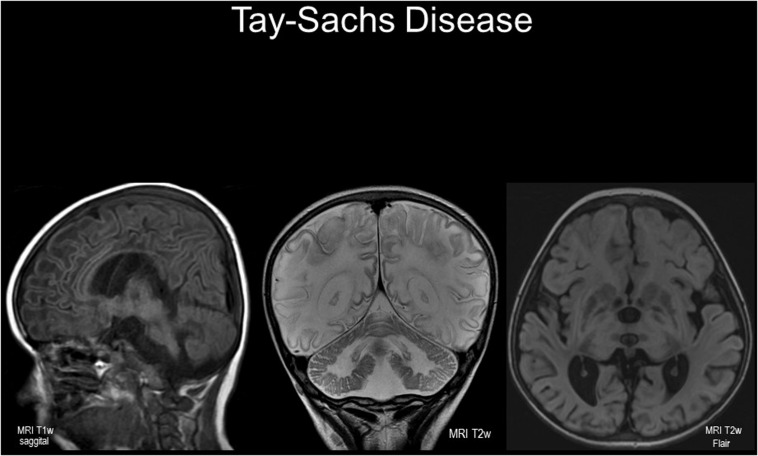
Cranial imaging in a 5 year-old child with infantile-onset GM2 gangliosidosis. Magnetic resonance images (left to right: sagittal T1w; coronal T2w; axial T2w FLAIR). Note the cerebral cortical and cerebellar atrophy with wide sulci and shrunken gyri; there are symmetrical diffuse white matter changes with reduced T1w intensity and hyper-intense signals in T2w images – the latter indicating demyelination. Abnormal signal intensity is seen in basal ganglia, peducles, and brain stem. Female infant born after normal delivery to unrelated white British parents without Ashkenazi ancestry; hypotonia noted at 7 months with cherry-red spots observed. Tay-Sachs disease confirmed enzymatically and by molecular analysis of the *HEXA* gene (well-known “severe” mutation frequent in British “Celtic” patients was identified). With scrupulous care and potentially salutary effects of a substrate-reducing agent (miglustat), the illness followed an unexpectedly protracted course. Nonetheless, characteristic startle responses to sounds, loss of motor skills, epilepsy and episodes of aspiration pneumonia led to deterioration with a peaceful death, aged 8 years.
